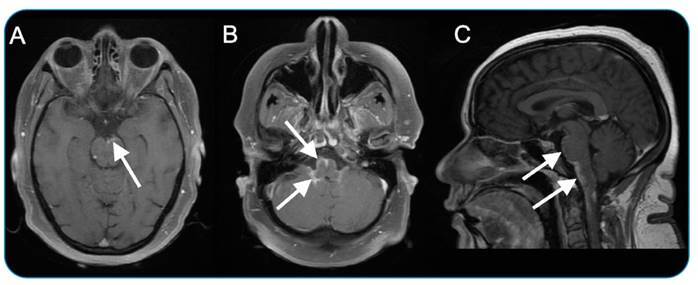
EBV meningitis. T1-weighted brain MRI with and without fat saturation after intravenous gadolinium injection (axial, A and B; sagittal, C) revealed abnormal leptomeningeal gadolinium enhancement in the basal cisterns, including the interpeduncular, pre-pontine, and pre-medullary cisterns, extending to the cervical spinal cord (arrows).
